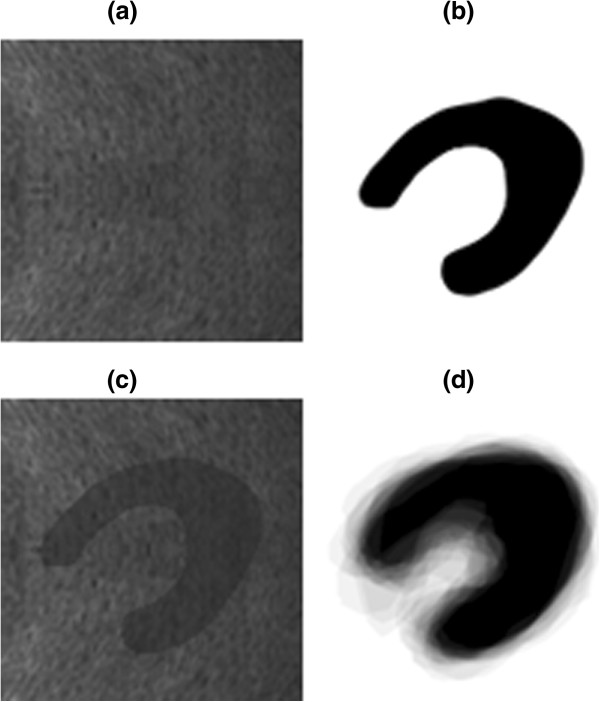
Synthetic image and initial shape model. (a) ultrasound noise image; (b) a shape come from the kidney shape space; (c) the mixture image of the noise image and shape image; (d) initial shape model.
